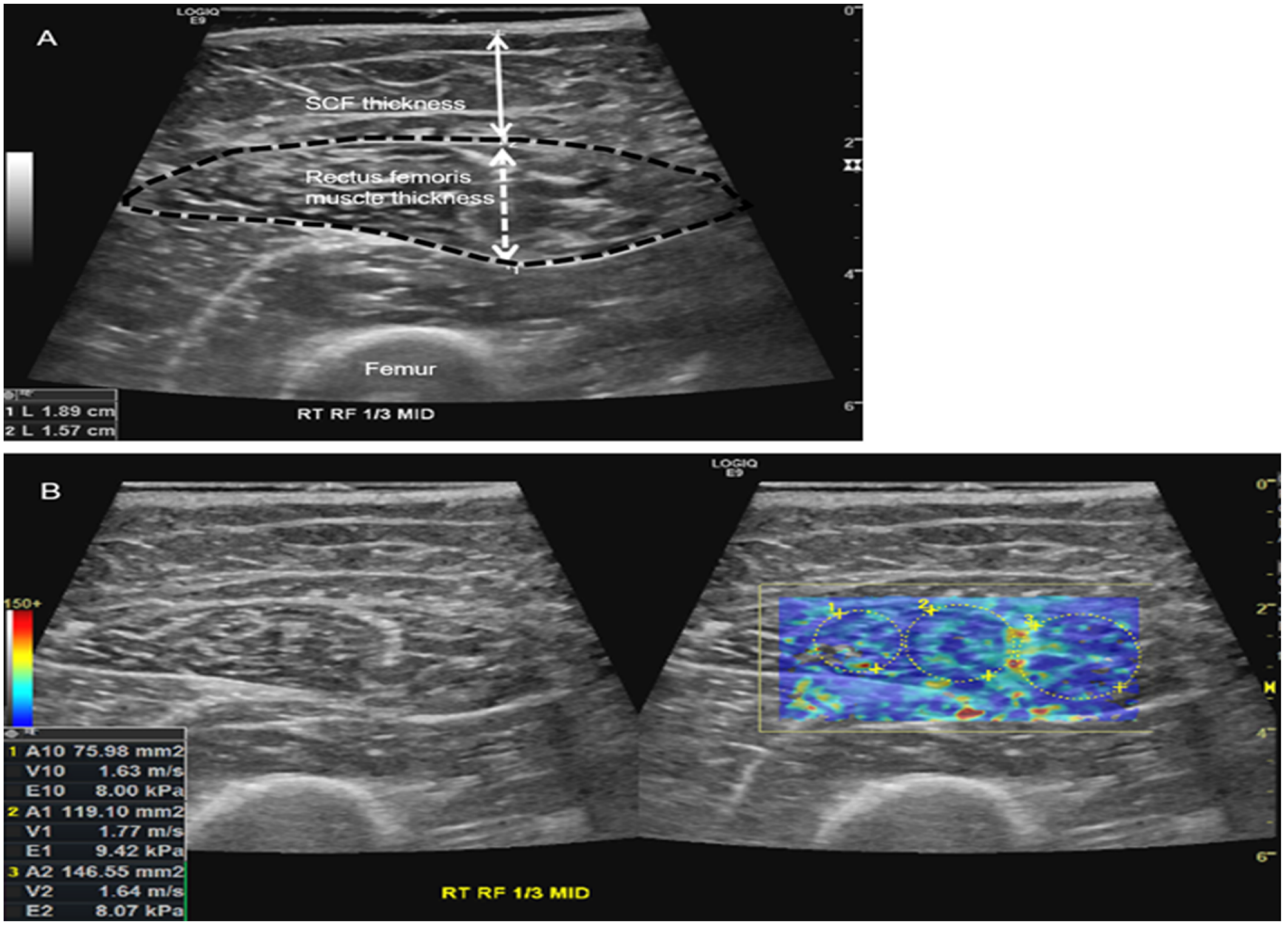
The representative image of ultrasonography measurements of mid rectus femoris muscle (RF). (A) On grayscale ultrasonography, the thickness of RF (white dashed double arrow), cross-sectional area of RF (black dashed line), and the thickness of subcutaneous fat layer (SCF) overlying RF (white double arrow) were measured. (B) On shear-wave elastography, the mean of shear wave velocity (SWV, m/sec) was measured.
